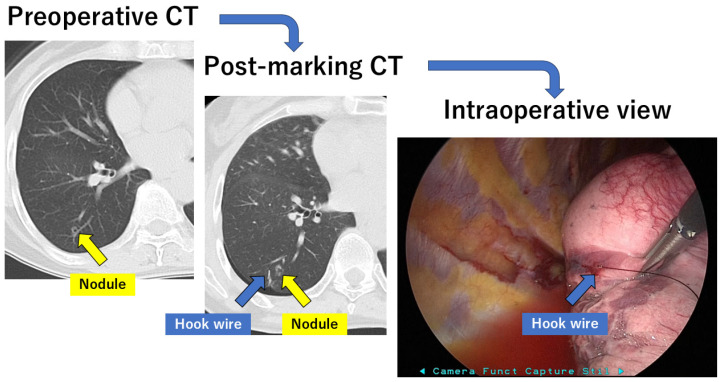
Preoperative marking with hook wire. CT: computed tomography.
